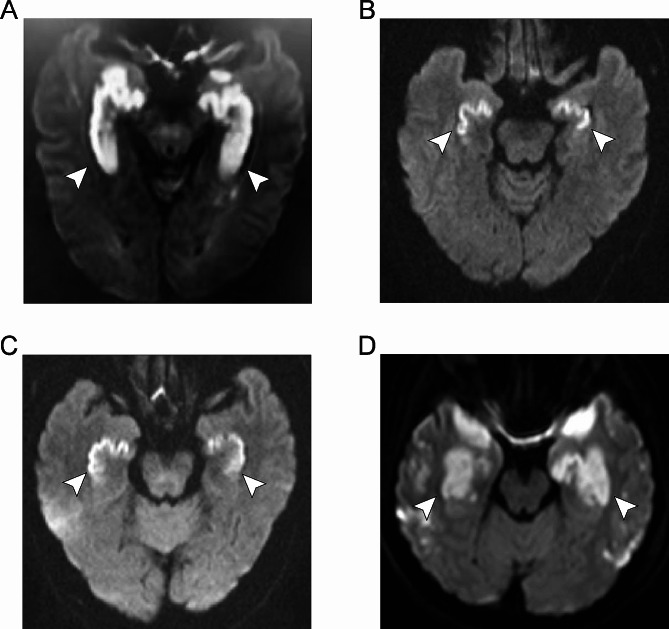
DWI obtained from four patients with acute carbon monoxide poisoning. (A) A 65-year-old man (patient A, COHb: 39%) examined at 48 h post-exposure, with demonstration of restricted diffusion (arrows) in the bilateral hippocampi. (B) A 61-year-old man (patient B, COHb: 19.2%) examined at 24 h post-exposure, with demonstration of restricted diffusion (arrows) in the bilateral hippocampi and globus pallidus. (C) A 62-year-old man (patient C, COHb: 7.8%) examined at 72 h post-exposure, with demonstration of restricted diffusion (arrows) in the bilateral hippocampi. (D) A 24-year-old woman (patient D, COHb: 7%) examined at 12 h post-exposure, with demonstration of restricted diffusion (arrows) in the bilateral medial temporal lobe and cerebral peduncle
